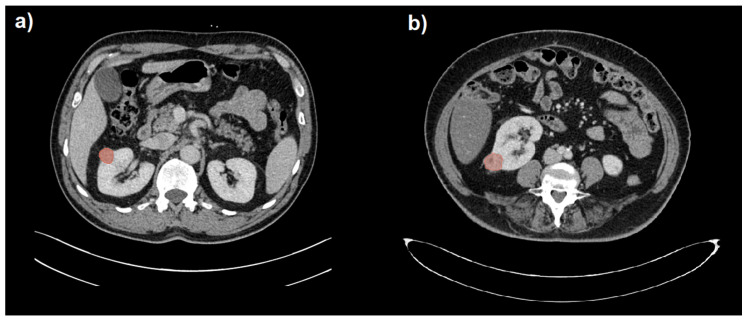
Segmentation (in red) of a benign (a) and a malignant (b) small renal mass (SRM) hardly distinguishable on portal venous phase CT images.
